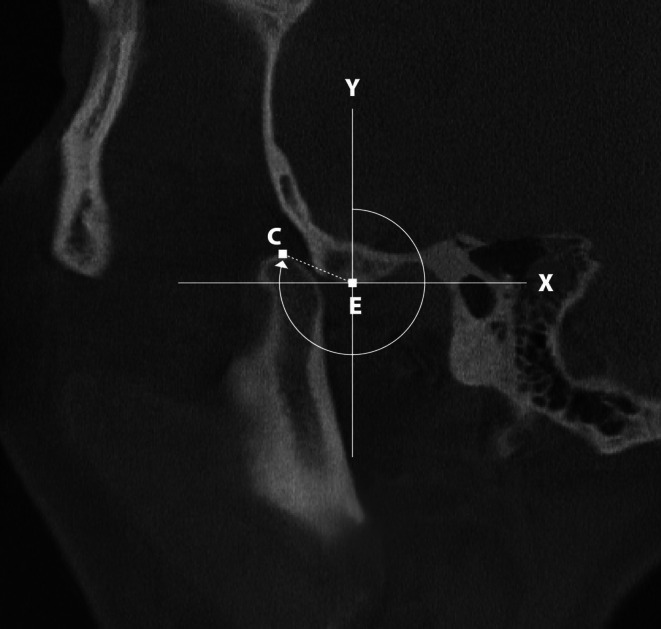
Measurement of the condylar angle (°) according to the method of Kalaykova et al. (2006), adapted for CBCT images. In the parasagittal slice, point E corresponds to the most inferior aspect of the articular eminence crest, and point C corresponds to the most superior aspect of the condylar cortex. A vertical line (*Y*‐axis) and its perpendicular horizontal line (*X*‐axis) were drawn from point E to establish a Cartesian coordinate system. The condylar angle was defined as the angle between the EC line and the vertical axis.
